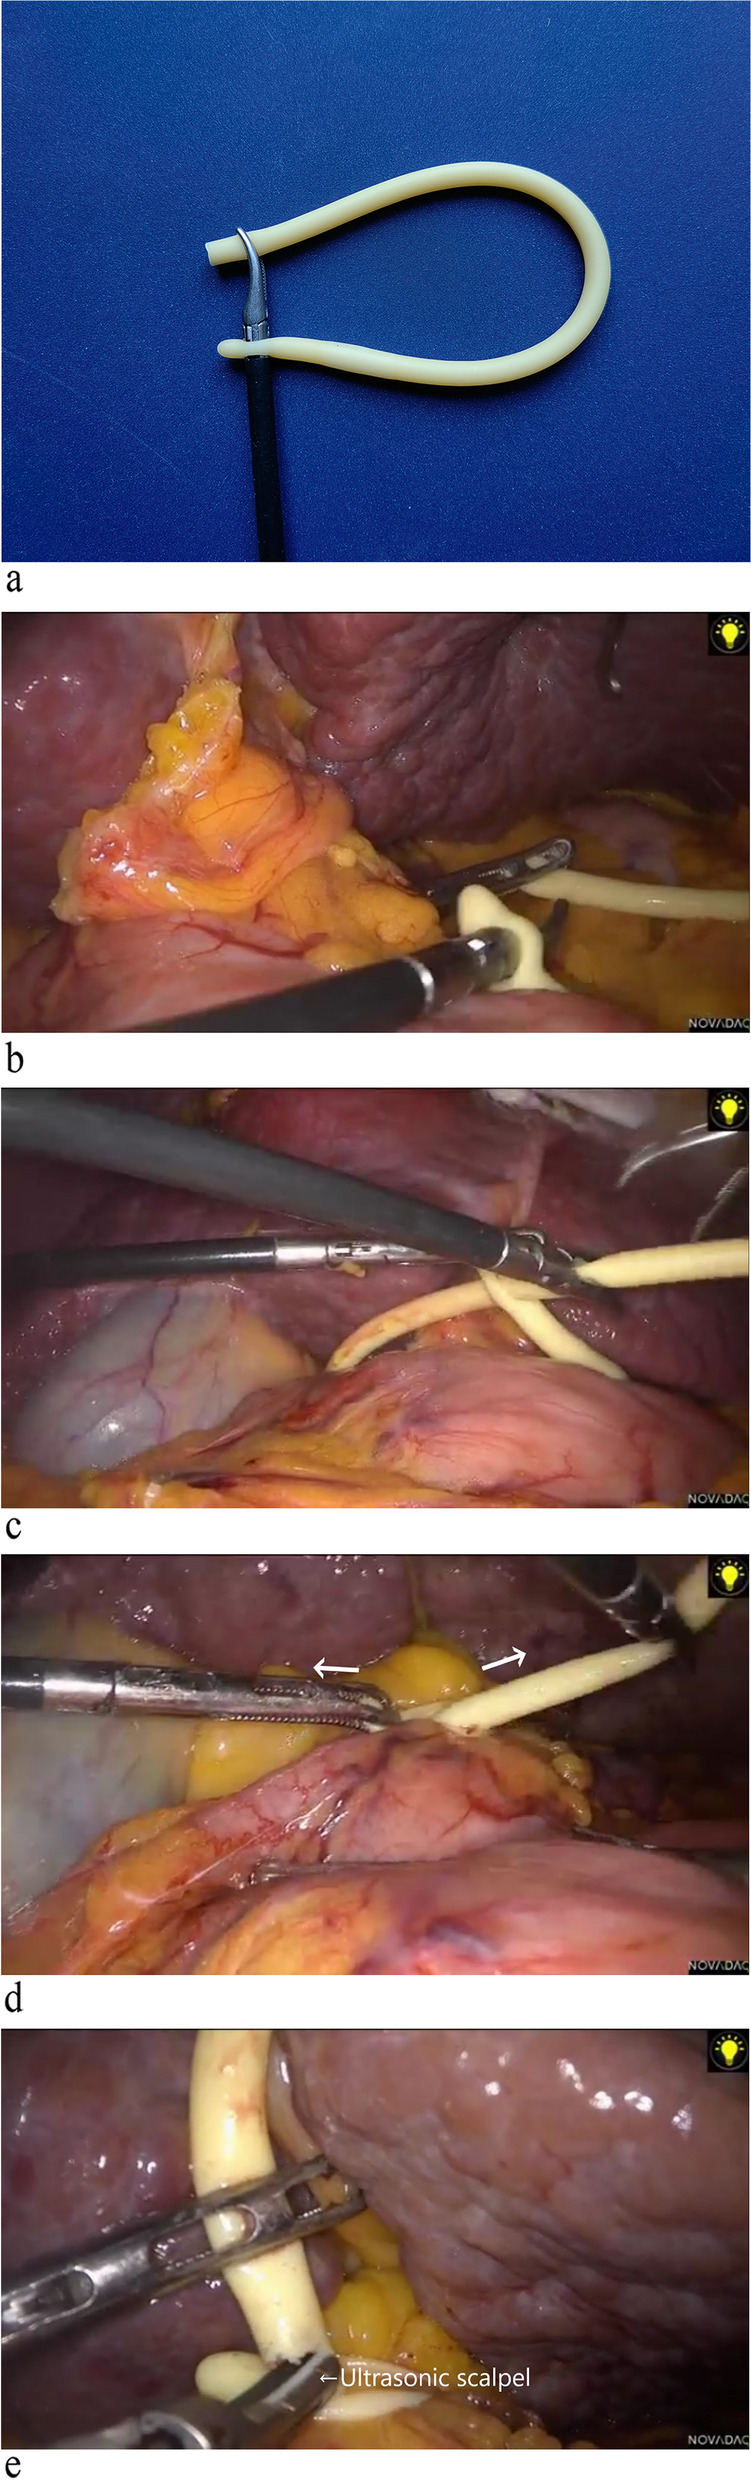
a Use the dissecting forceps to pass through the small hole in the front end of the catheter and clamp the tail of the catheter. b The operator’s left-hand grasper guides the catheter through the Winslow hole. c Form a loop around the hepatoduodenal ligament. d Tighten the loop with both hands in the direction of the arrow. e Cut about 1/2 or 1/3 of the circumference of the catheter body at the junction of the catheter loop and the catheter body with an ultrasonic scalpel
